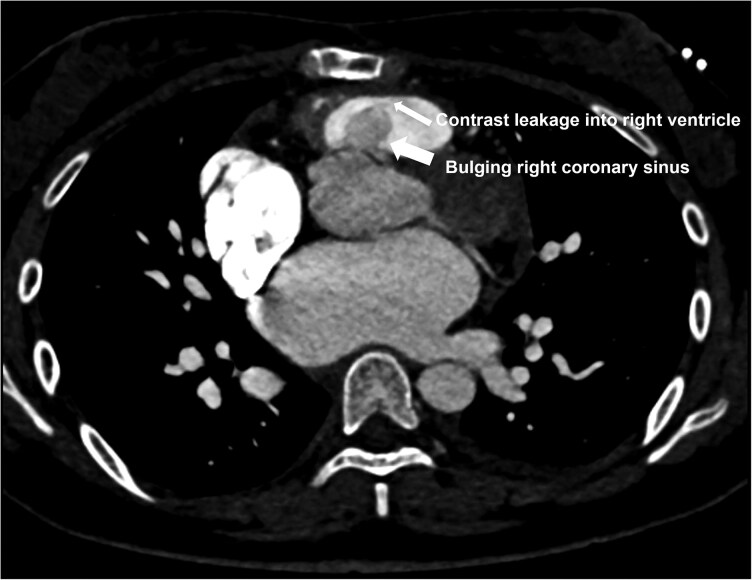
Cardiac computed tomography angiography (CTA) demonstrating rupture morphology. CTA shows bulging of the right coronary sinus towards the right ventricular outflow tract with contrast leakage into the right ventricle (white arrows), consistent with a ruptured right sinus of Valsalva aneurysm.
